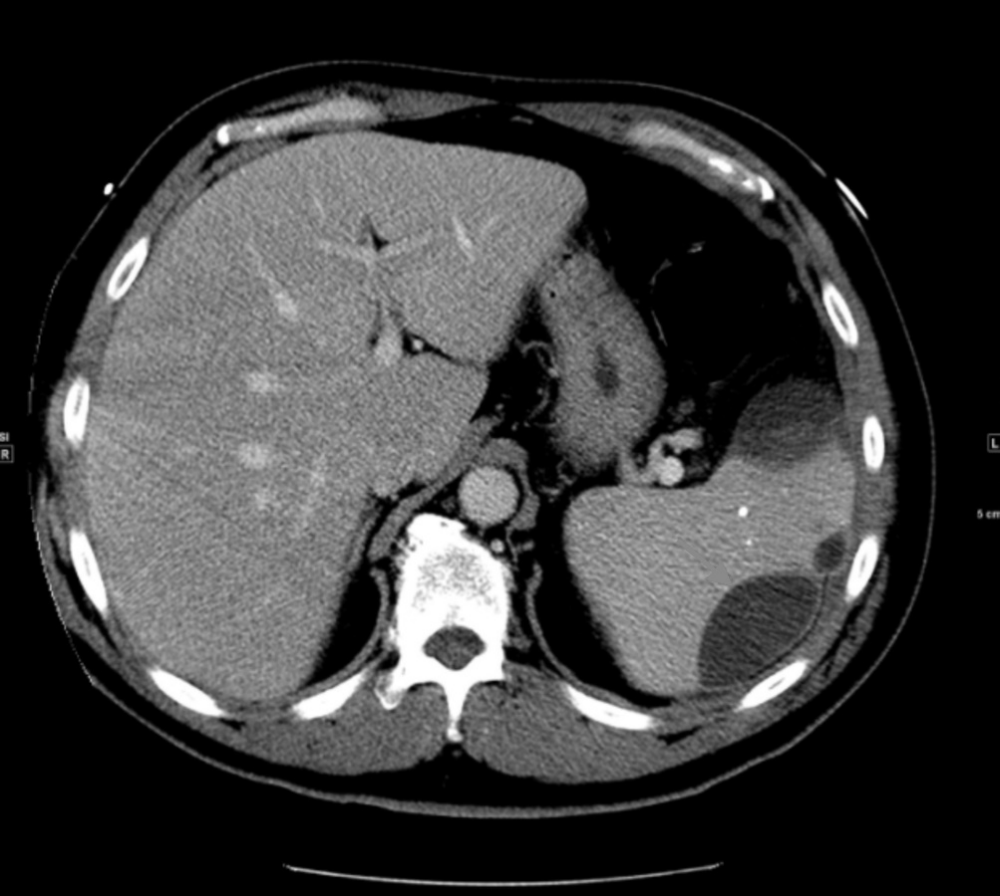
Splenic Abscess A computed tomography (CT) of the chest and abdomen demonstrated a splenic fluid collection measuring 4.3 cm x 2.8 cm, clinically suspicious for an abscess.
